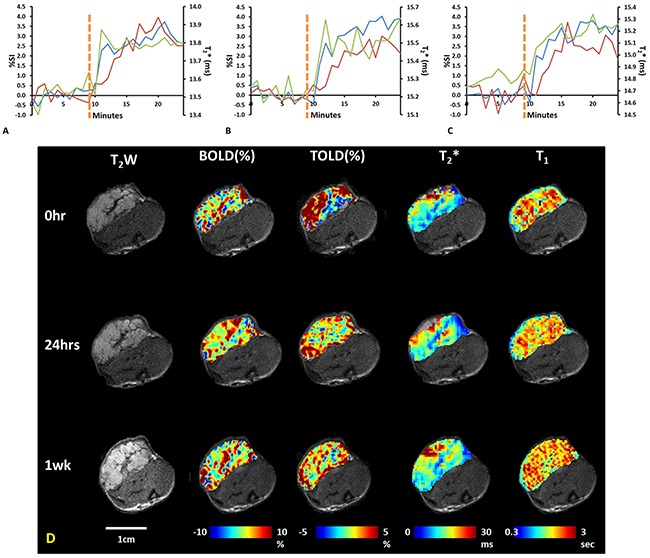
Oxygen sensitive MRI of a representative control A549 lung tumor Upper graphs: BOLD (blue), TOLD (red) and T_2_* (green) curves show response to oxygen breathing challenge of a control tumor at three time points: (A) baseline, (B) 24 hours, and (C) 1 week. Yellow dotted line indicates transition from air to oxygen breathing. (D) T_2_w images of tumor (0.7 cm^3^) and overlaid parametric response maps showing %ΔSI of BOLD and TOLD, T_2_* map and T_1_ map (left to right) at baseline, 24 hours and one week.
